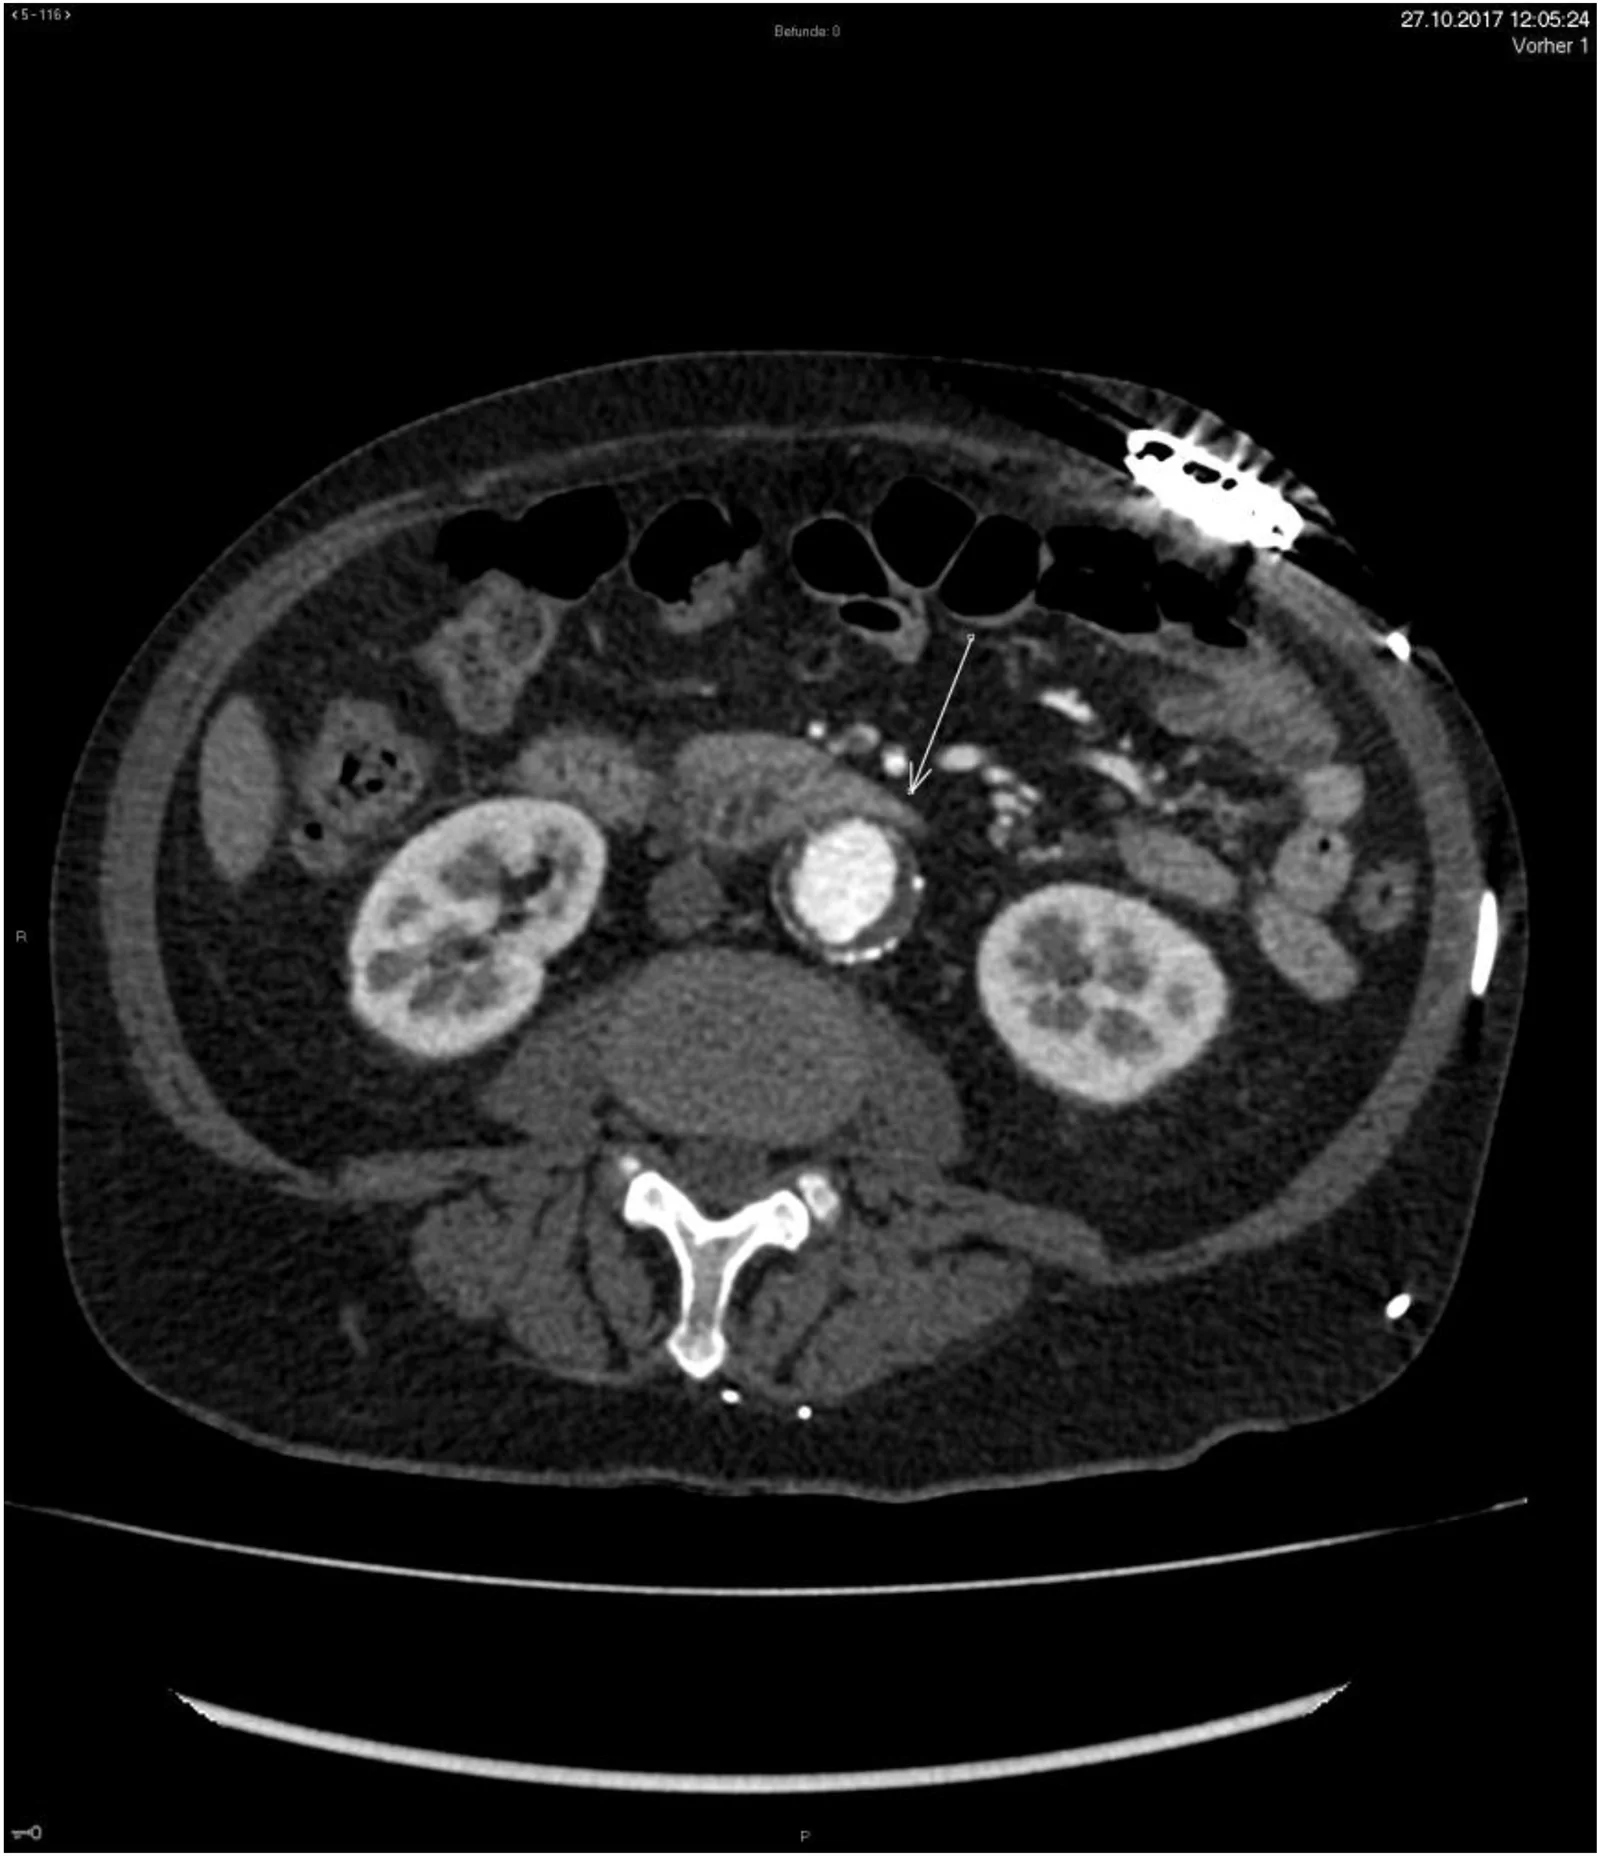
Retrospective axial contrast-enhanced CT image from 2017 demonstrating focal narrowing of the third portion of the duodenum adjacent to the infrarenal abdominal aortic aneurysm (arrow). No convincing upstream duodenal dilatation was present, and the imaging findings were insufficient to establish a diagnosis of clinically significant duodenal obstruction at that time.
